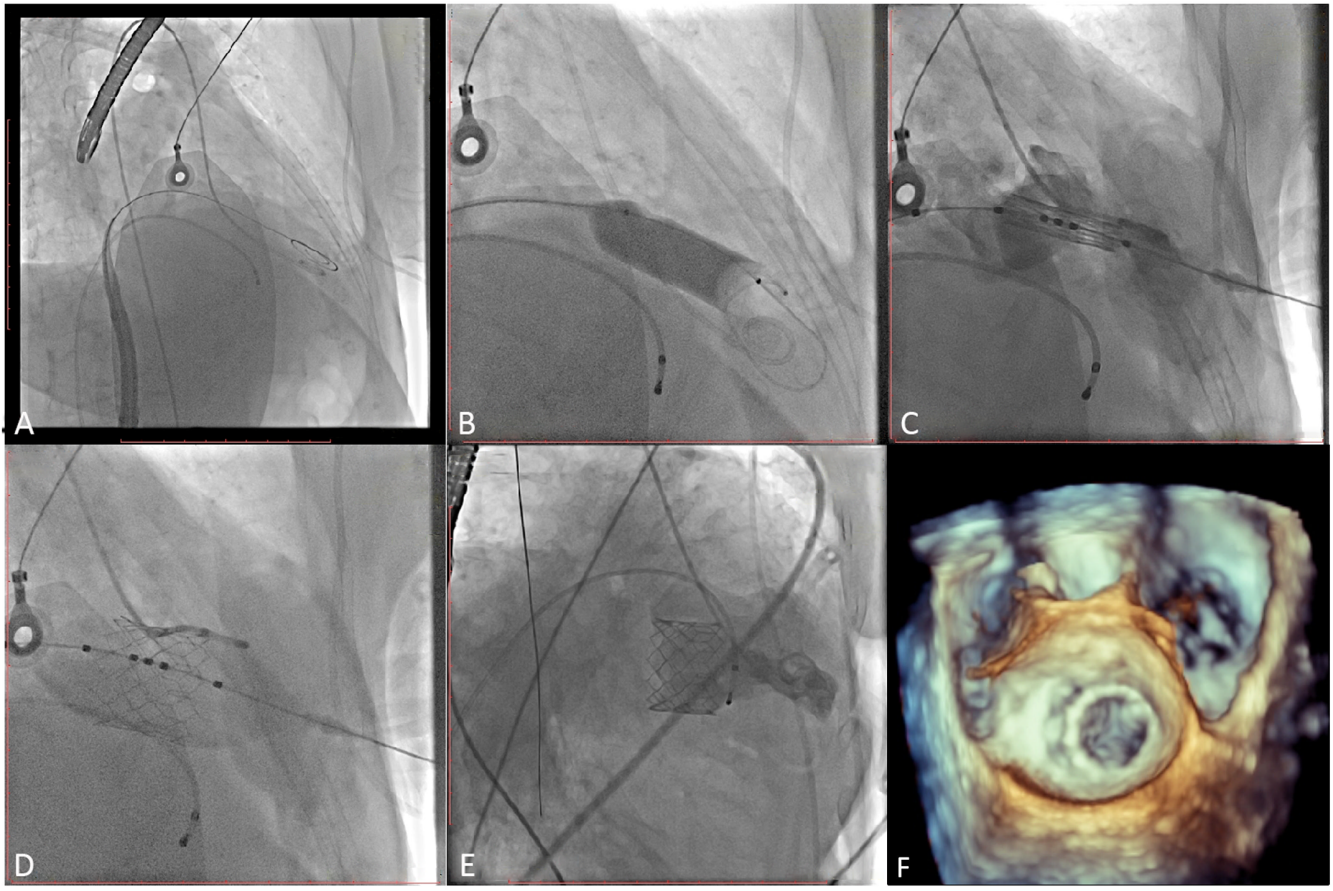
Procedural details. (A) The pigtail catheter was inserted into the left ventricle. (B) Pre-dilation was used before delivery system insertion. (C) The prothesis was positioned at mitral valve. (D) The prosthesis was completed release and post-dilation. (E, F) Digital subtracted angiography and transesophageal echocardiography displayed the good position and function of the prosthesis.
